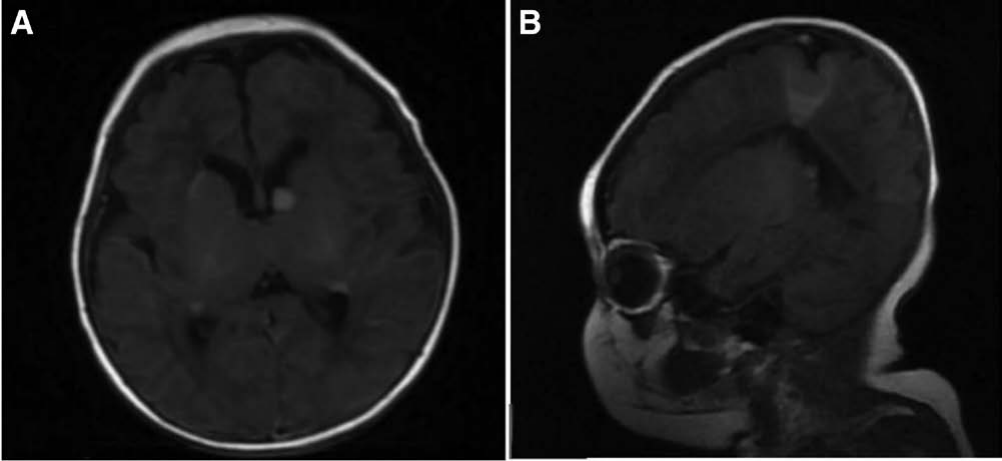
The head MRI showed that there were many spot-shaped abnormal signal shadows under the ependymal of bilateral lateral ventricles, T1 was high, T2 was low; the left parietal cortex is locally thickened, showing patchy T1 uneven high signal, and DWi slightly high signal.
